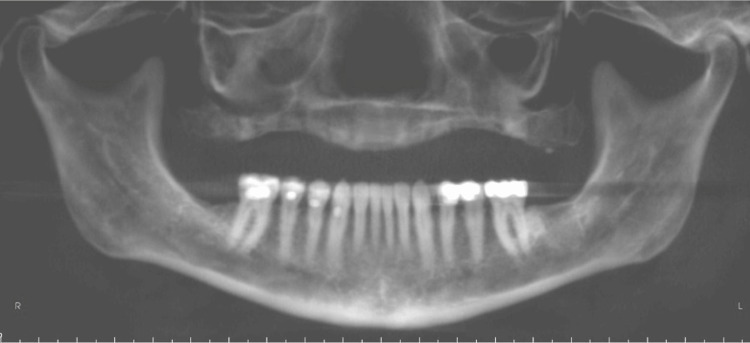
CBCT reconstructed panoramic by Software B
